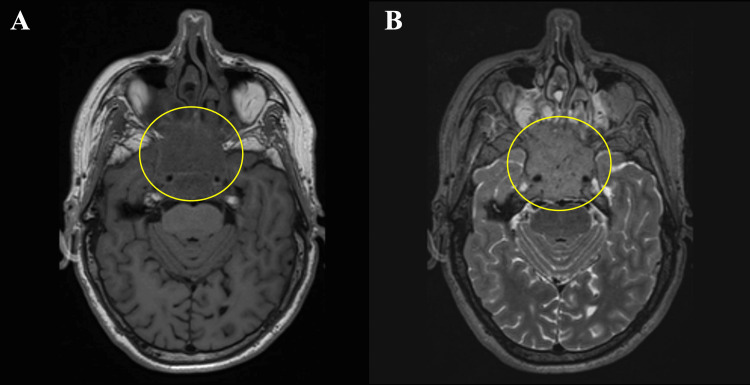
A: Tumor image in yellow circles on T1-weighted magnetic resonance imaging, B: tumor image in yellow circles on T2-weighted magnetic resonance imaging.
